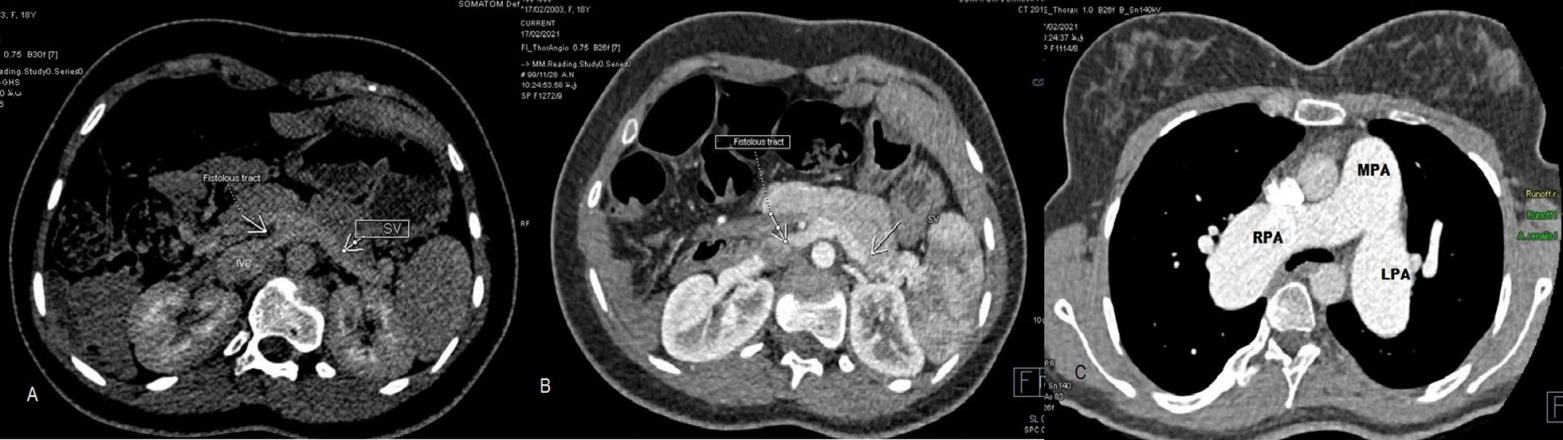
Contrast-enhanced axial CT scan in porto-venous phase demonstrating abnormal fistulous tract directly connecting splenic vein (SV) and superior mesenteric vein to inferior vena cava with absent main portal vein (A, B). Axial contrast CT image showing dilated pulmonary artery in favor of significant pulmonary hypertension (C)
